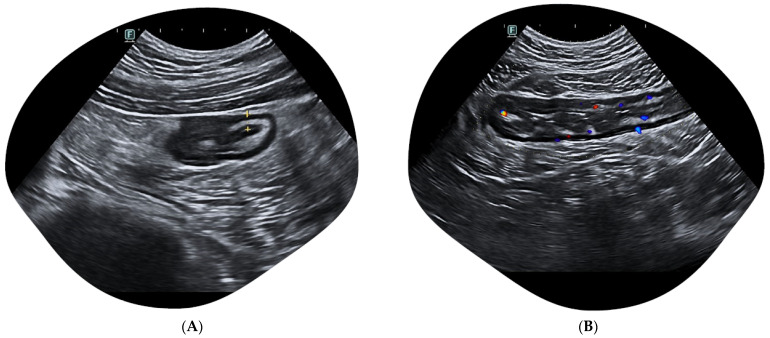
Ultrasound presentation of an active left-sided ulcerative colitis. (A) Increased BWT (3.4 mm) in a mild active left-sided ulcerative colitis, in the descending colon. (B) Severe acute ulcerative pancolitis: the sigmoid colon results severely inflamed with a CDS 3 according to Limberg score, complete disruption of BWS (hyperechoic spots in the mucosa/submucosal interface suggesting deep ulcerations) and increased BWT.
